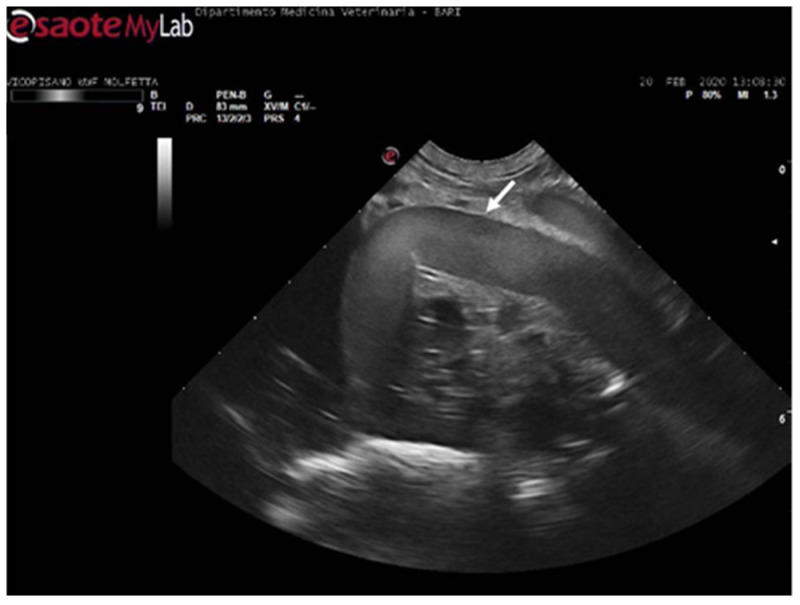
Ultrasound scan from the left prefemoral window reveals normal vascular flow within the iliac artery (the white arrow indicating its wall).
